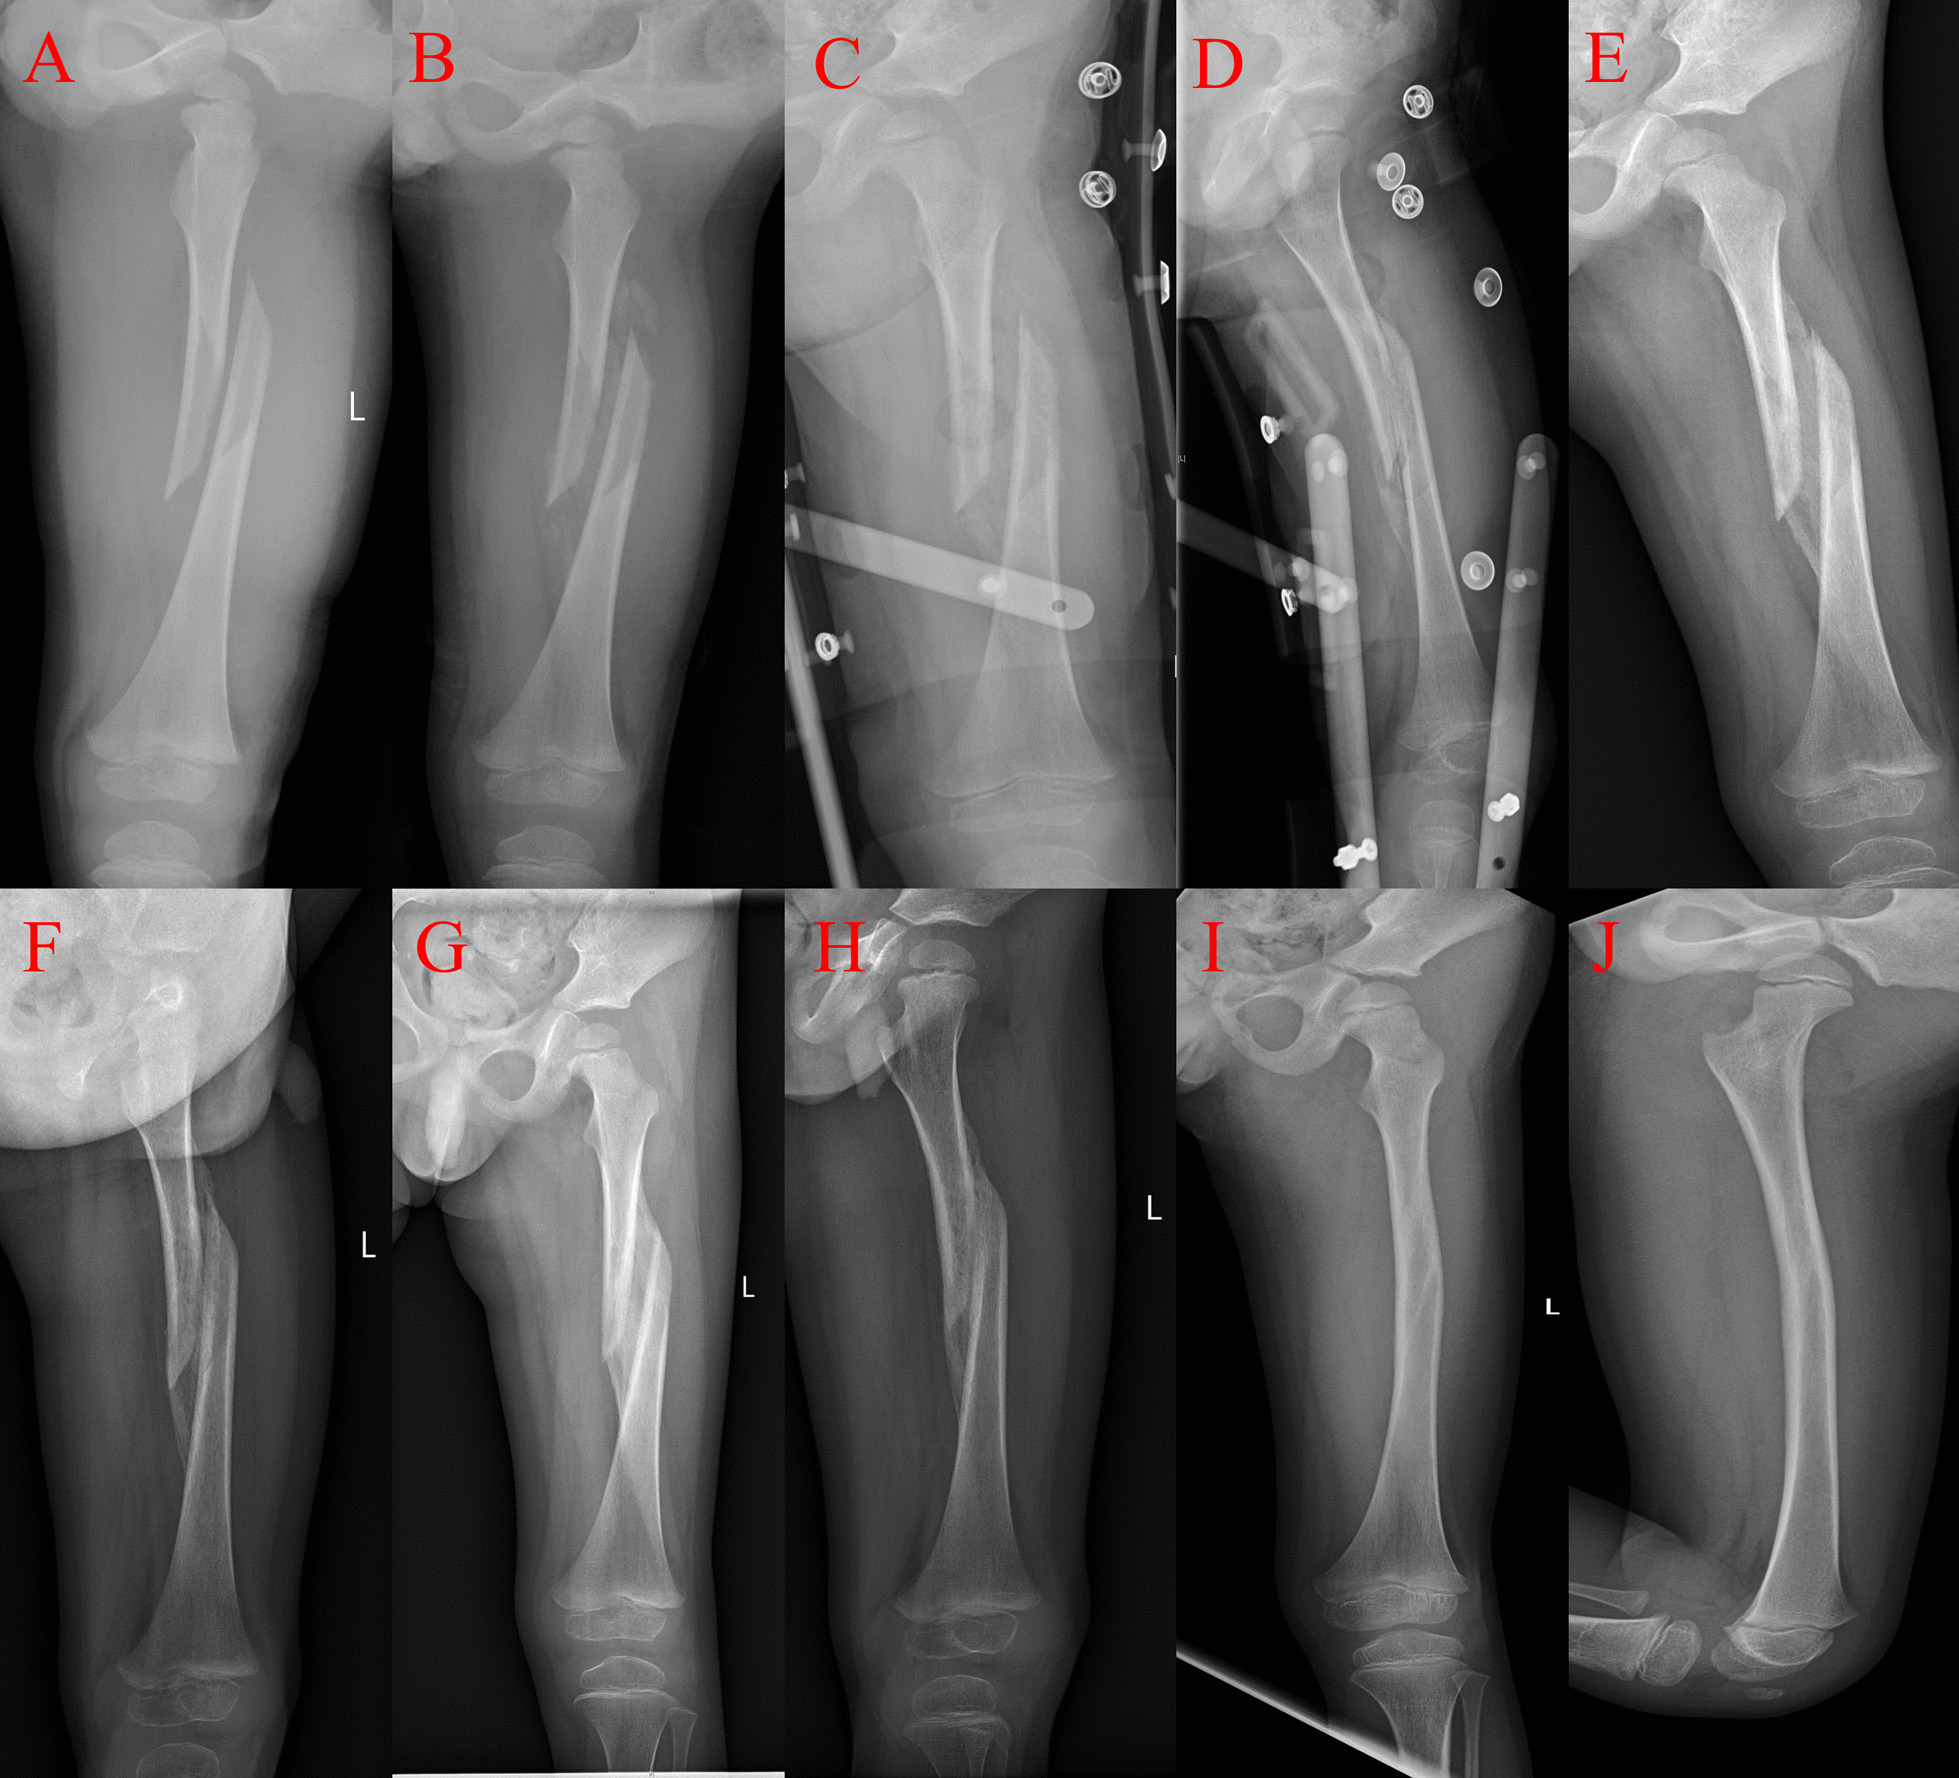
A 3-year-old male injured after a fall was treated using skin traction combined with a spica brace. A AP radiograph of the left femoral fracture by skin traction on day 8. B Bone callus as observed on AP radiograph by skin traction and then, spica brace on day 15. C, D Bone callus progressed further on AP and lateral radiographs. The spica brace is still visible on day 24. E, F AP and lateral radiographs show femur fracture remodeling at 3 months postoperatively. G, H AP and lateral radiographs show femur fracture remodeling at 4 months postoperatively. I, J AP and lateral radiographs show femur fracture remodeling at 15 months postoperatively
